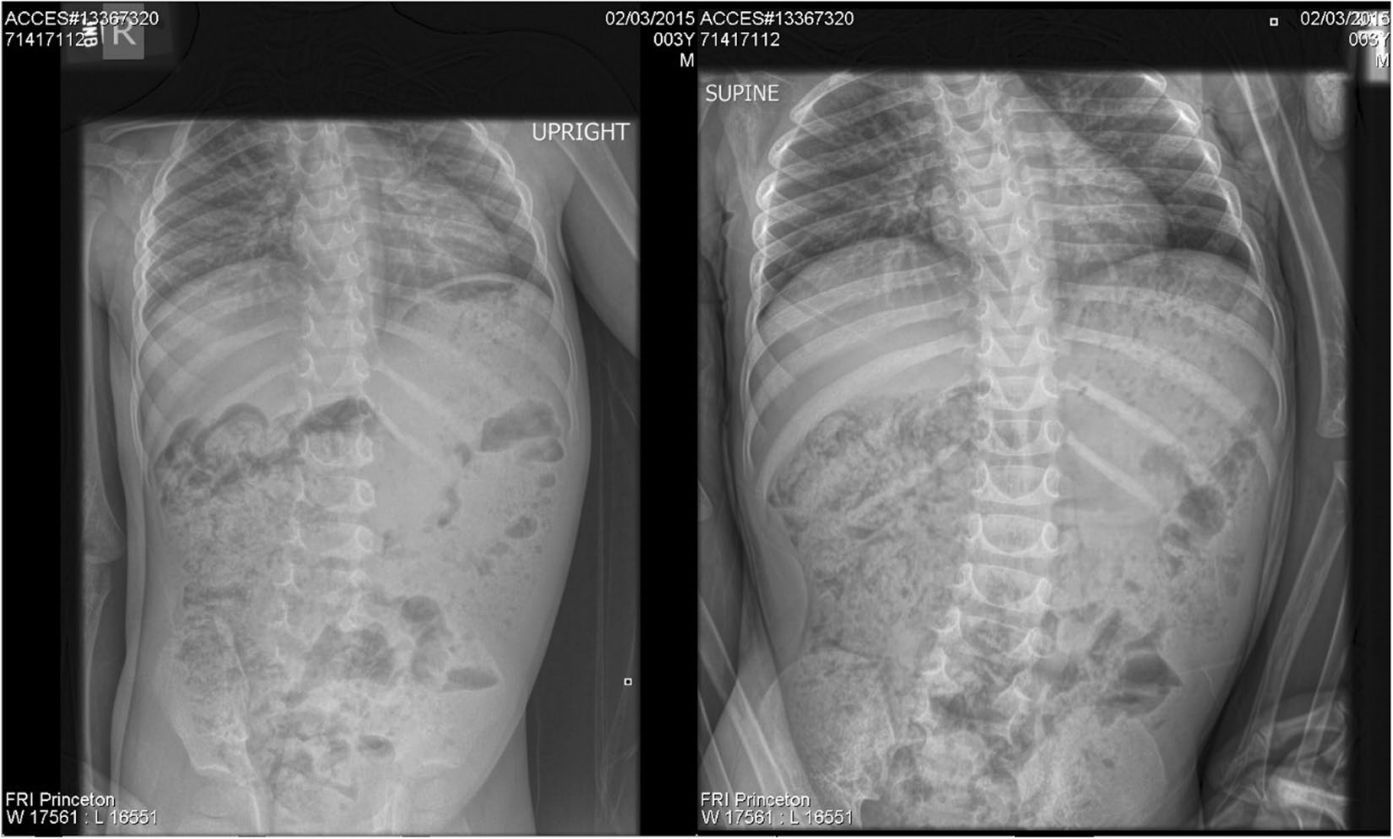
Abdominal radiograph of 3 year old boy with autism and right-sided colonic fecal loading with colonic distention.
